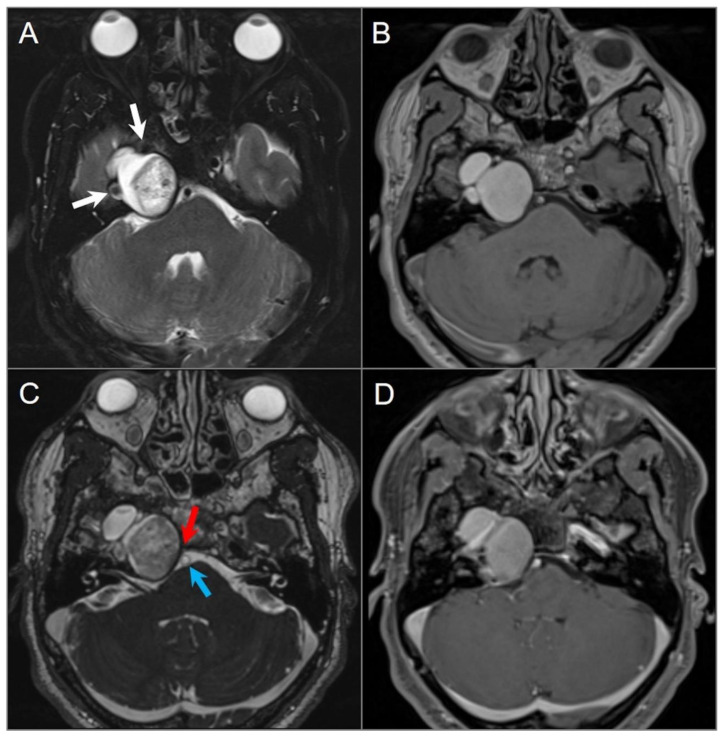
Cholesterol granuloma. (A) Axial fat-suppressed T2W and (B) noncontrast T1W images show a lobulated, expansile lesion in the right petrous apex with intrinsic high signal intensity on T1 and mixed signal intensity with smooth hypointense margins on T2. The lesion is in close proximity with the petrous ICA (white arrow). (C) Axial CISS image shows extension of the lesion into the right cerebellopontine angle with mild mass effect on the pons and abutment of the basilar artery (blue arrow). The lesion effaces right Dorello’s canal, where there is mass effect with effacement of the cisternal segment of the right sixth cranial nerve (red arrow). (D) Axial fat-saturated postcontrast T1-weighted image demonstrates no significant enhancement of the lesion, although this is difficult to evaluate due to intrinsic T1 hyperintensity.
